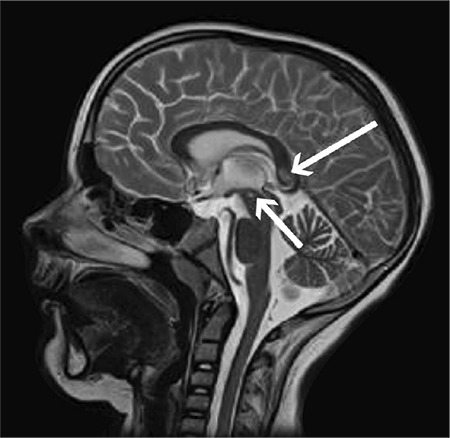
Brain magnetic resonance imaging. T1-weighted scan. Lesions located in the corpus callosum and the midbrain
